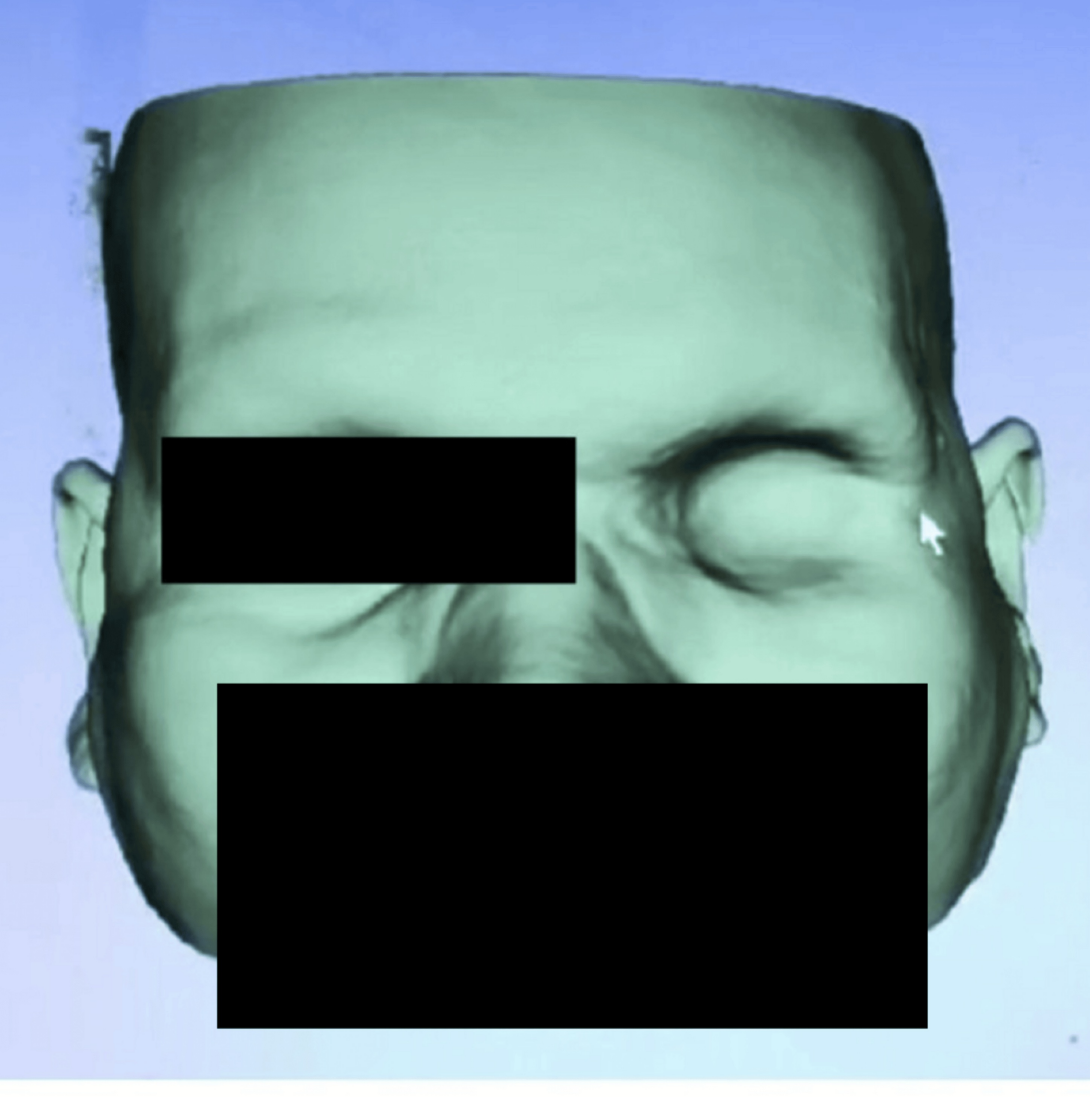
Digitally obtained impression of the left orbit using CT scan.
